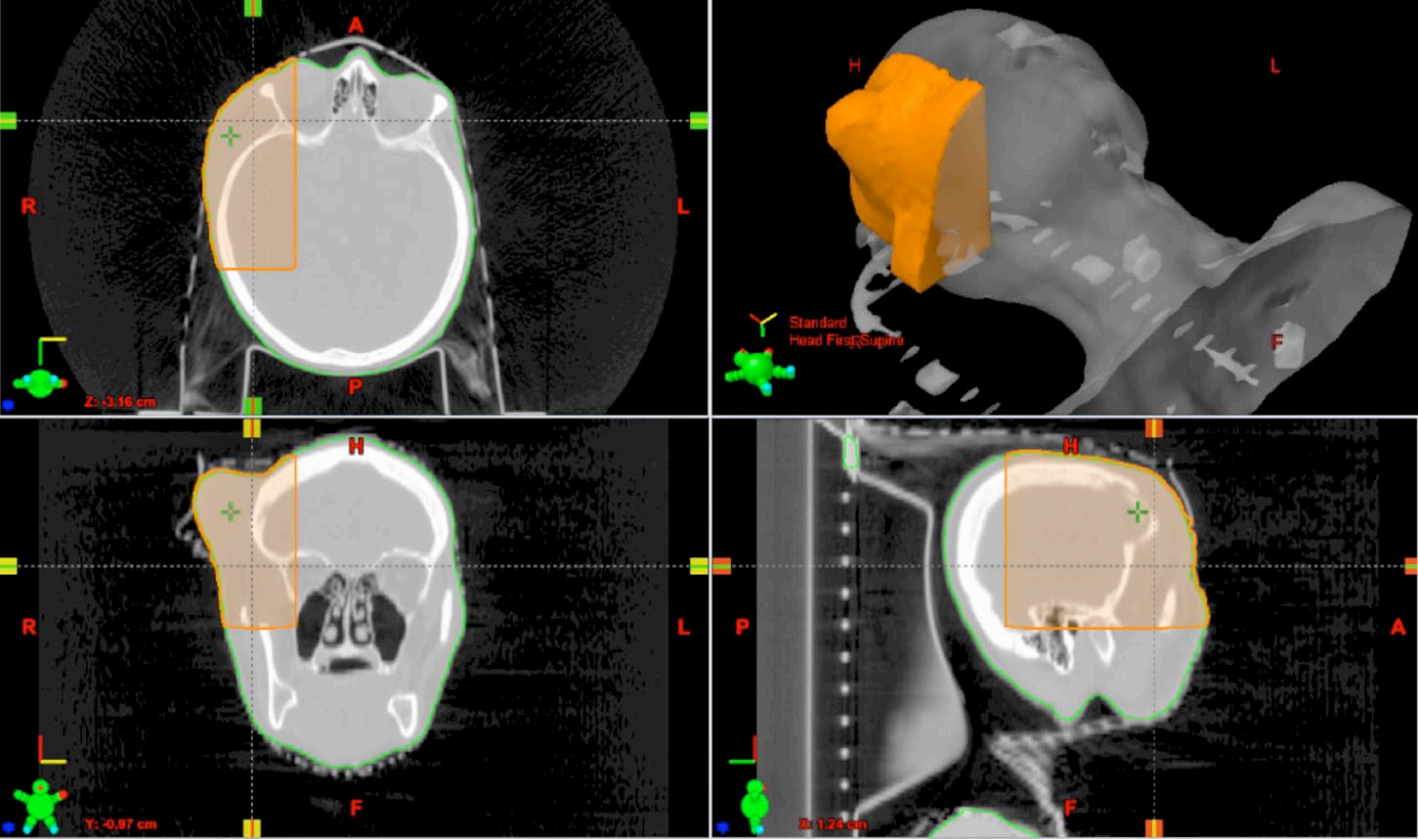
The process of designing a positive mold of a patient and printing for Aquaplast bolus fitting. Axial, coronal, and sagittal views from the CT of our patient are shown counterclockwise from the top left. The area highlighted in orange was the area sent to the 3D printer. A 3D rendering is seen in the top right corner.
